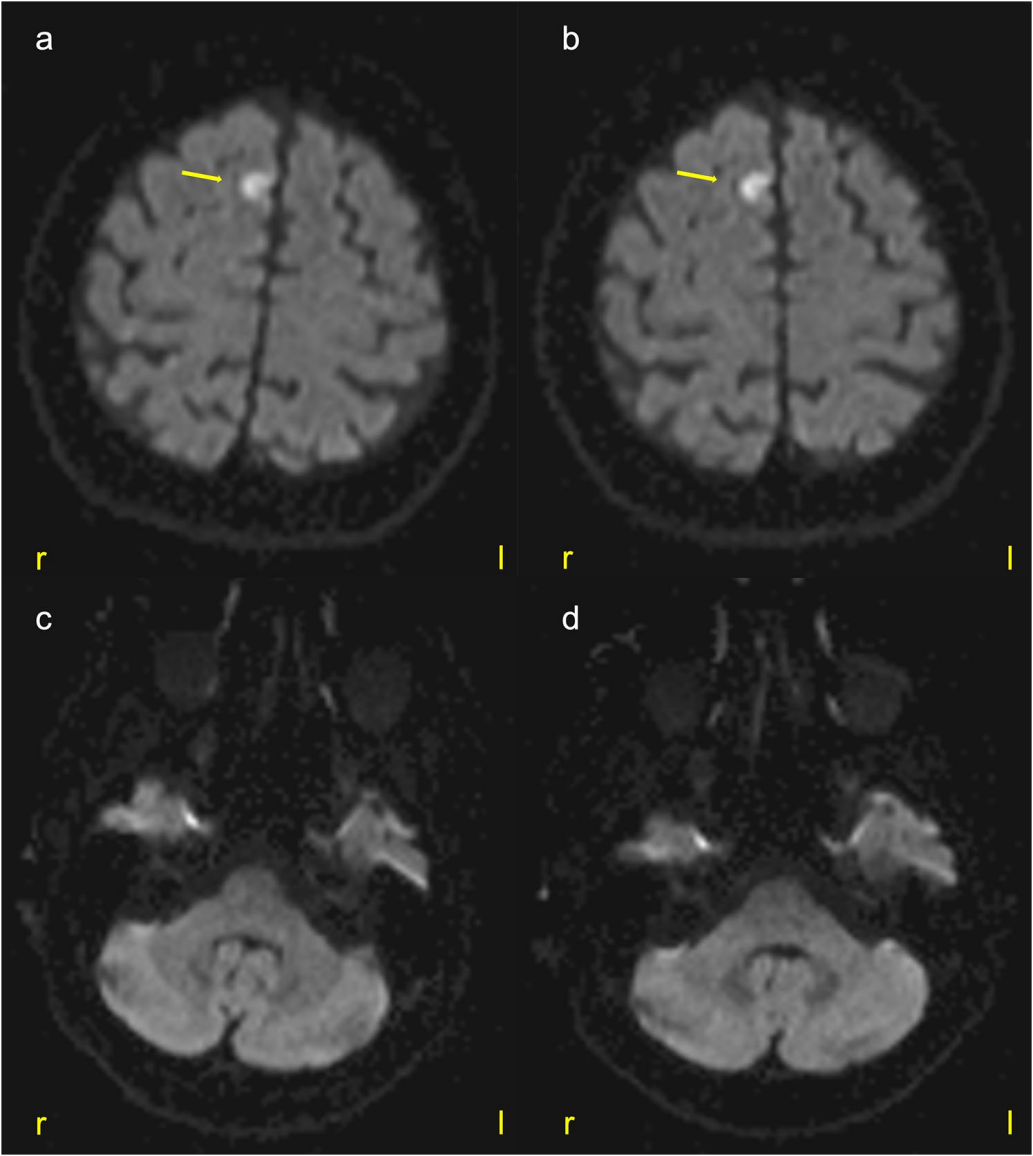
MRI findings of the brain parenchyma. Despite the extended perfusion delay in PWI (see Fig. 2a, b), only a small ischemic lesion can be detected in the right anterior territory (yellow arrow) in diffusion-weighted imaging (DWI). In the right posterior circulation (vessel narrowing of the right vertebral artery, see Fig. 2c), there was neither evidence of perfusion delay (not shown) nor any diffusion disturbance. (a, c) DWI at the time of PWI, (b, d) DWI 4 h later
